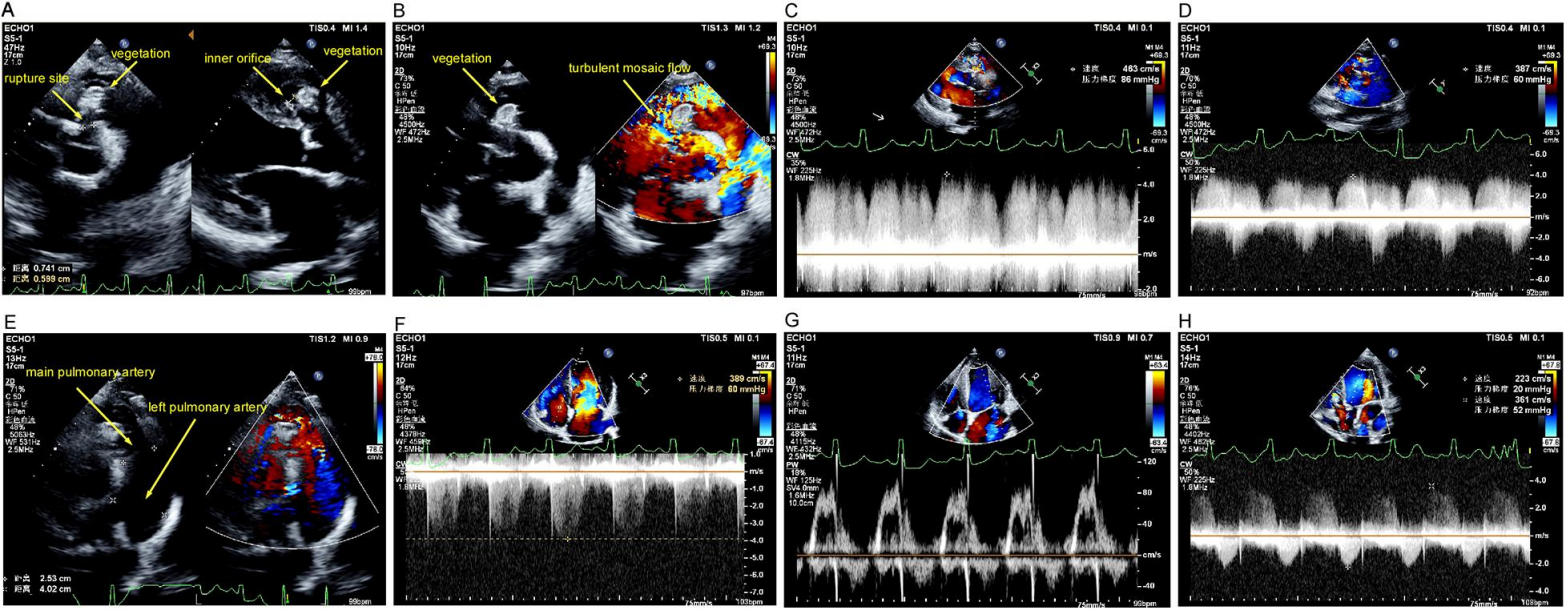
Preoperative transthoracic echocardiographic findings. (A) Parasternal short-axis and long-axis views showing a saccular outpouching of the right coronary sinus of Valsalva bulging into the infundibulum of the right ventricular outflow tract. The aneurysm wall appears echogenic, with a mass-like echo attached to its apex. A distinct rupture point is visible at the aneurysm neck; the inner orifice and rupture site measure approximately 0.60 and 0.74 cm in diameter, respectively. (B) Parasternal short-axis view with color Doppler demonstrating turbulent mosaic flow at the sinus of Valsalva aneurysm, within the right ventricular outflow tract, and into the pulmonary artery. (C) Parasternal short-axis view with continuous-wave Doppler detecting a biphasic shunt flow signal across the aneurysm rupture site, with a peak velocity of 463 cm/s and a pressure gradient of 86 mmHg. (D) Parasternal short-axis view with color Doppler showing turbulent mosaic shunt signals at the ventricular septum near the aneurysm base. Continuous-wave Doppler reveals a peak velocity of 387 cm/s and a pressure gradient of 60 mmHg. (E) Parasternal short-axis view revealing significant dilation of the main pulmonary artery (2.53 cm) and the left pulmonary artery (4.03 cm). (F) Apical four-chamber view with continuous-wave Doppler showing mild tricuspid regurgitation. The peak regurgitant velocity is 389 cm/s, estimating a tricuspid regurgitant pressure gradient (TRPG) of 60 mmHg. (G) Apical four-chamber view with color Doppler demonstrating mild to moderate mitral regurgitation. (H) Apical five-chamber view with color Doppler showing mild aortic regurgitation. The accompanying antegrade aortic flow is accelerated, with a maximum velocity of 223 cm/s, corresponding to a pressure gradient of 20 mmHg. RCSVA, right coronary sinus of Valsalva aneurysm; RVOT, right ventricular outflow tract; TRPG, tricuspid regurgitant pressure gradient; LVEDD, left ventricular end-diastolic dimension; LA, left atrial; RV, right ventricular; LVEF, left ventricular ejection fraction.
